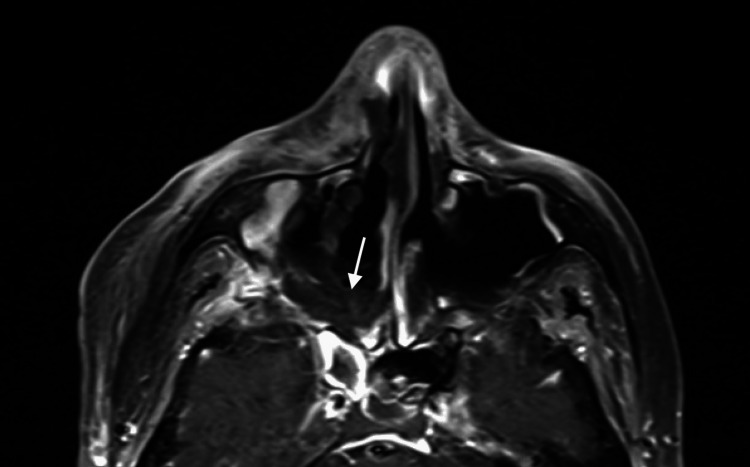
Post-operative day nine axial MRI sinus Paranasal sinus necrosis (white arrow) and multiple phlegmons were seen on imaging, resulting in the patient returning to the OR for repeat debridement.
